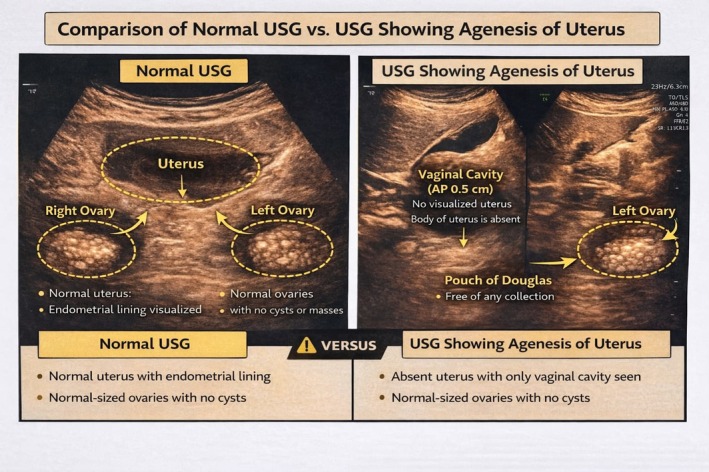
Comparison of normal pelvic ultrasonography and ultrasonography showing Müllerian agenesis. Side‐by‐side ultrasonographic comparison of pelvic anatomy. (a) Normal pelvic ultrasonography demonstrates a well‐defined uterine body with a visible endometrial echo centrally, along with normally positioned bilateral ovaries. (b) Ultrasonography in Müllerian agenesis, showing non‐visualization of the uterine body with identification of a linear structure representing the vaginal cavity (anteroposterior diameter approximately 0.5 cm). Bilateral ovaries are visualized and appear normal in size and echotexture, and the pouch of Douglas is free of any collection.
